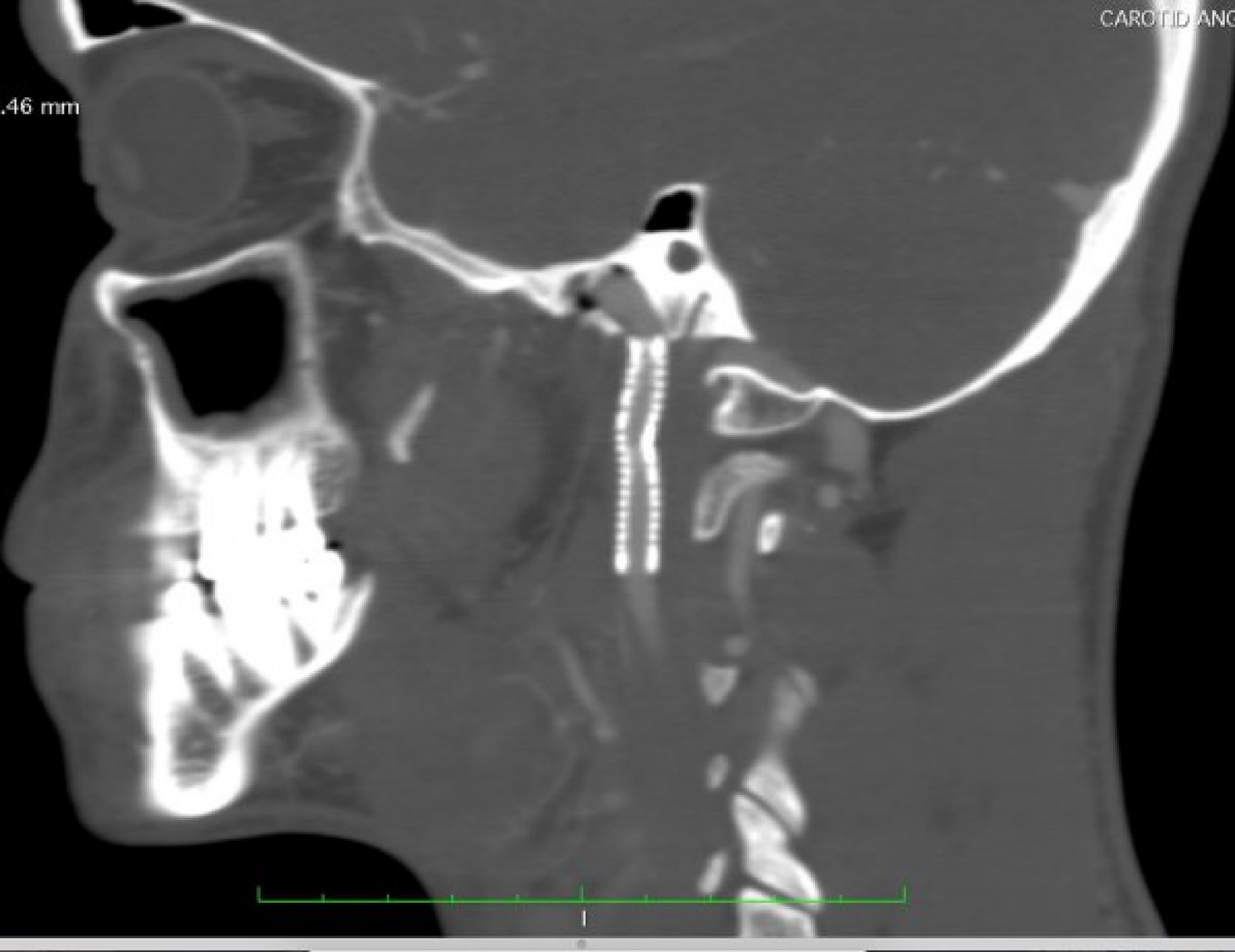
One‐month postoperative CTA revealing patent stent‐graft.
